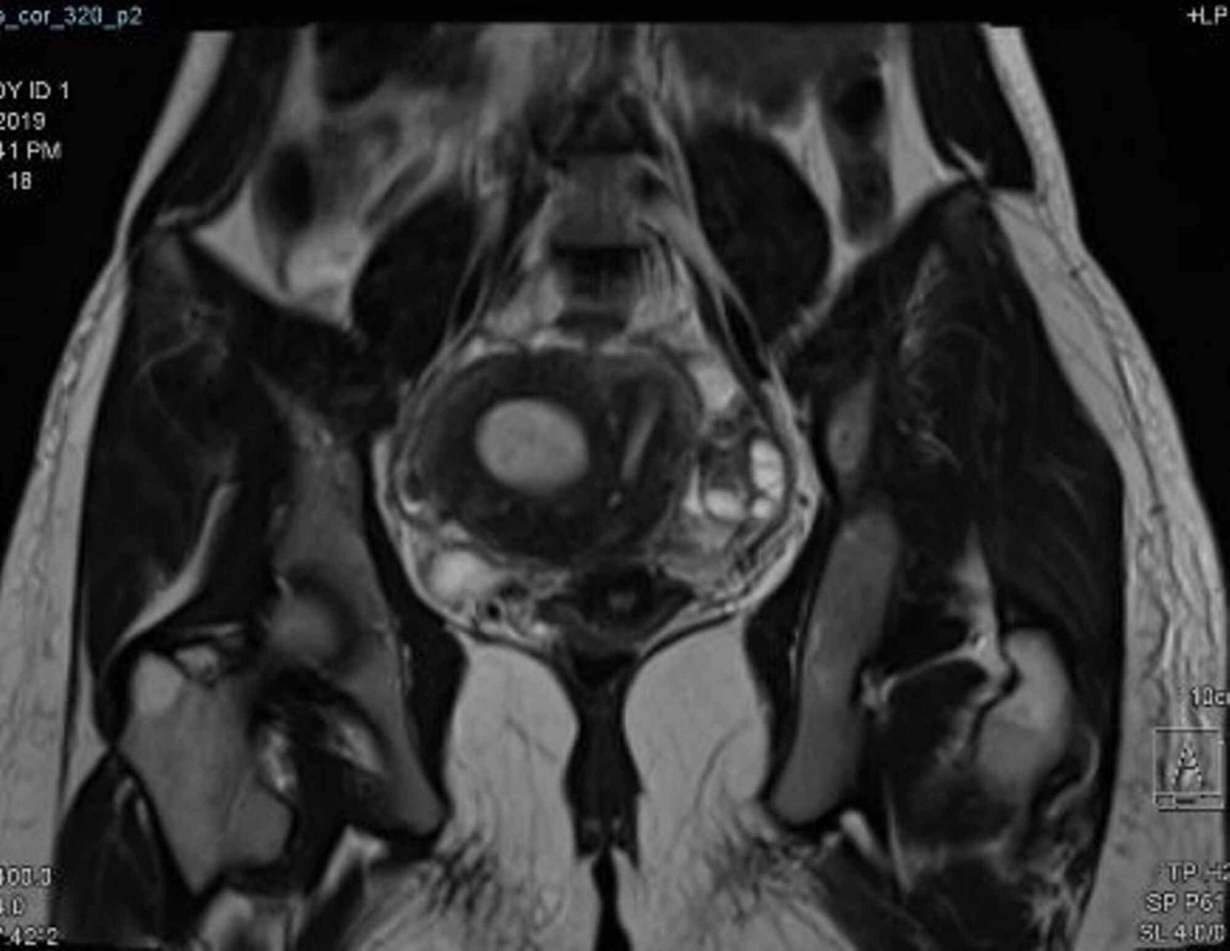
MRI of the pelvis Pelvic MRI: T2 signal MRI indicated the presence of the right-side functional rudimentary horn with hematometra in a unicornuate uterus. Both ovaries were demonstrated MRI: magnetic resonance imaging
